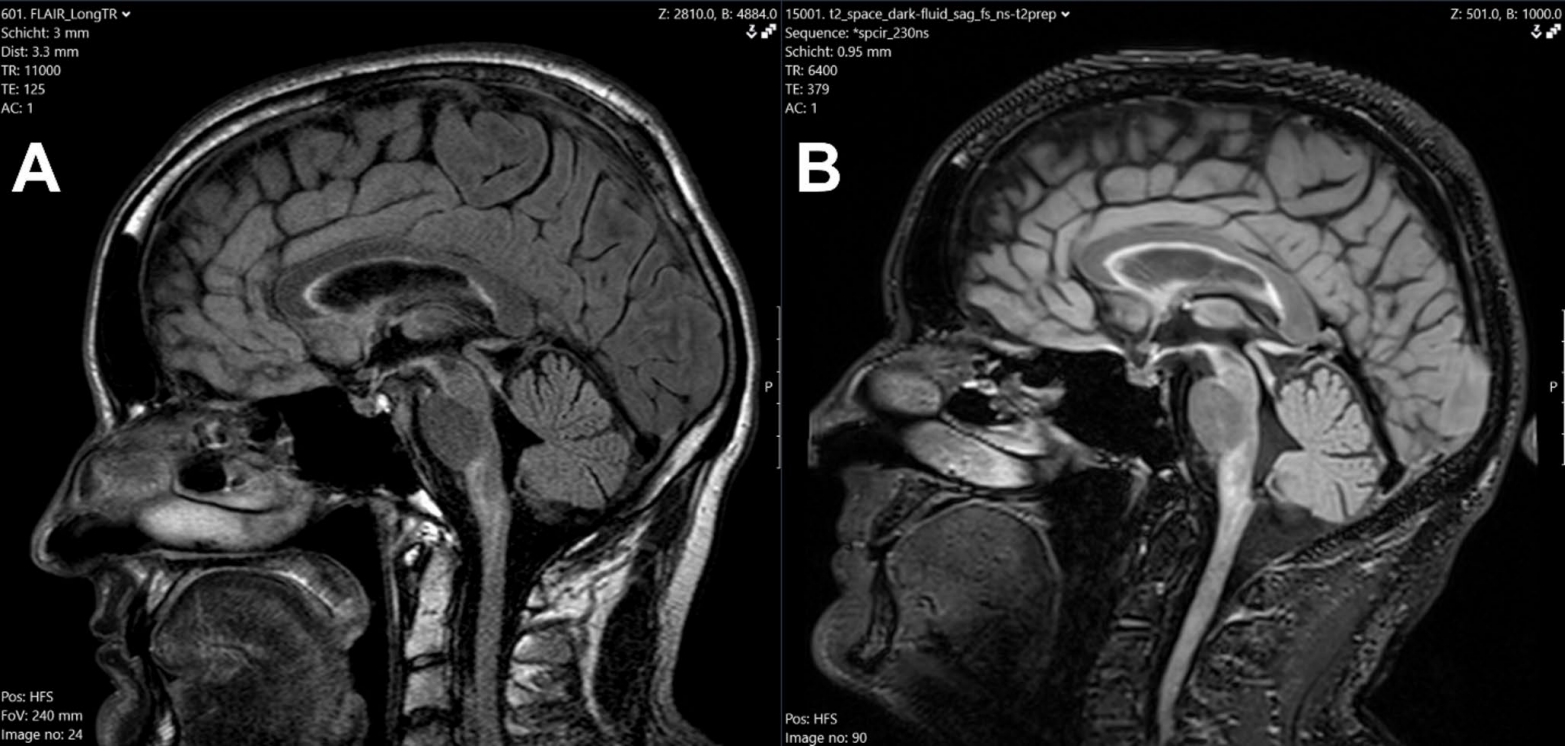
MRI findings (FLAIR) at the age of 32 (A) compared to the findings at the age of 45 (B)
